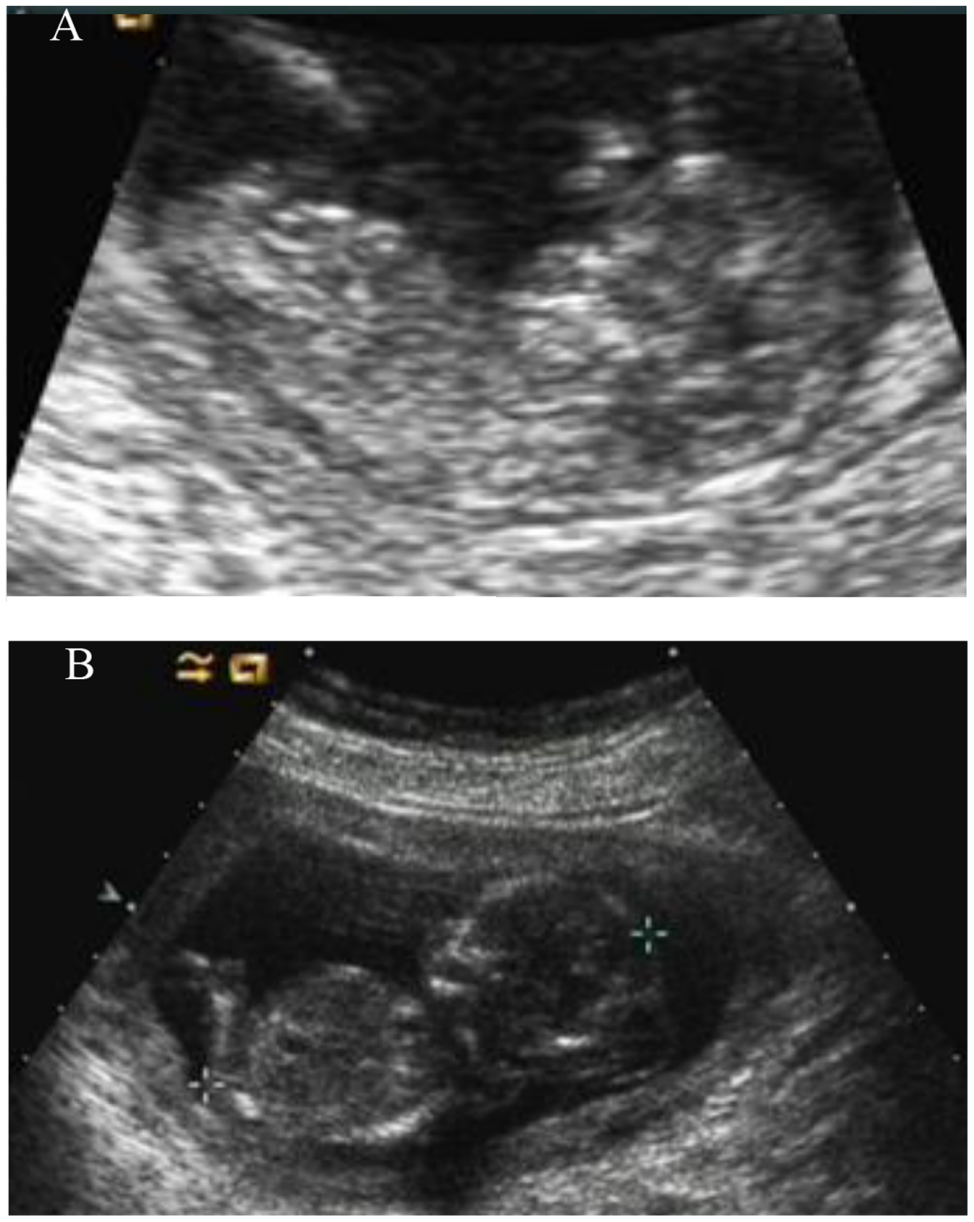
Ultrasound image of the normal and abnormal fetuses. A: The ultrasound image of PGD fetus at the 12 weeks’ gestation showing intact skull and did not have an enlarged abdomen. B: The ultrasound image of the third pregnancy of this couple at the 14 weeks’ gestation showing occipital encephalocele and enlarged abdomen.
